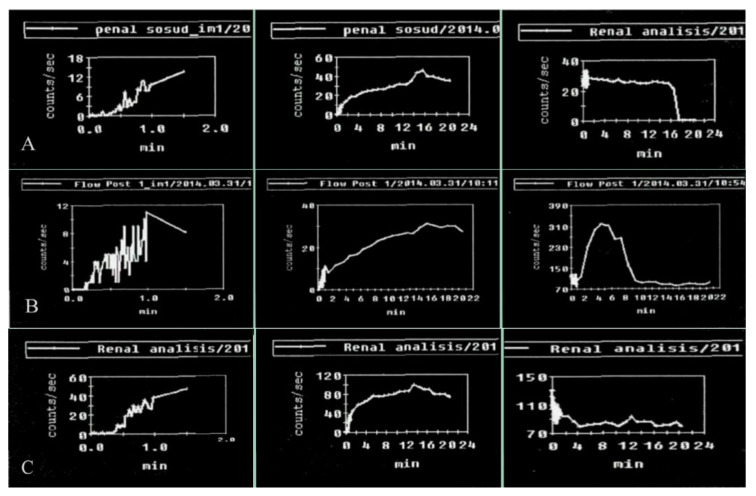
Results of penile scintigraphy in the visualization of vasculogenic erectile dysfunction: (A) arterial insufficiency; (B) venous insufficiency; (C) microcirculation disorder.
